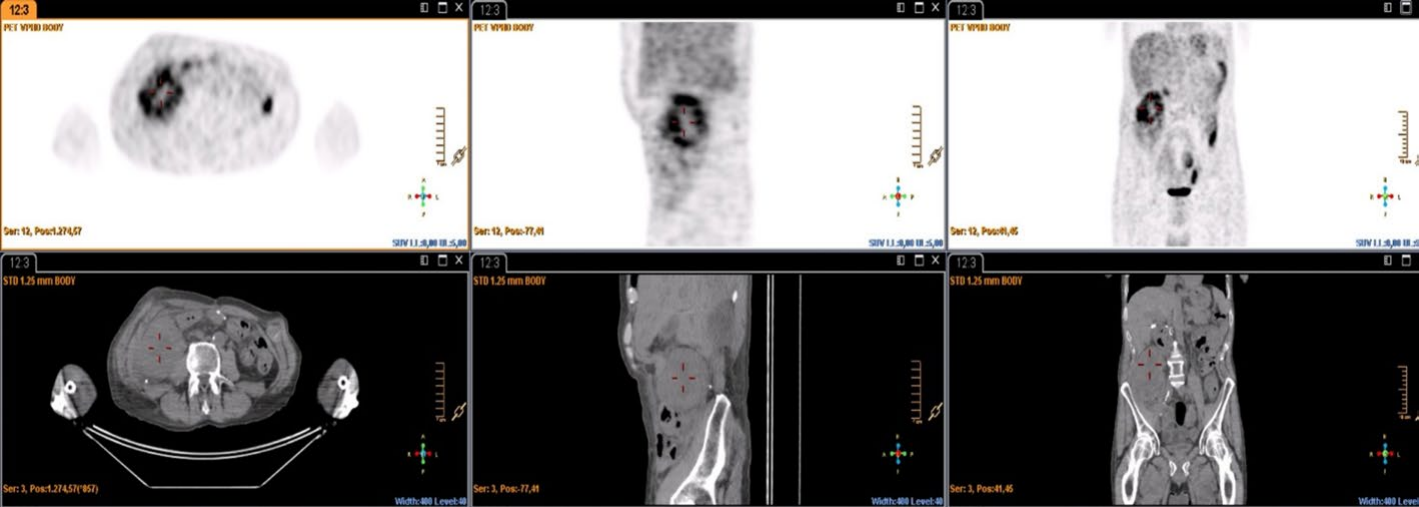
64-year-old male with high-grade spindle cell sarcoma. PET CT showed a mesenteric mass with heterogeneous ^18^F-FDG uptake
